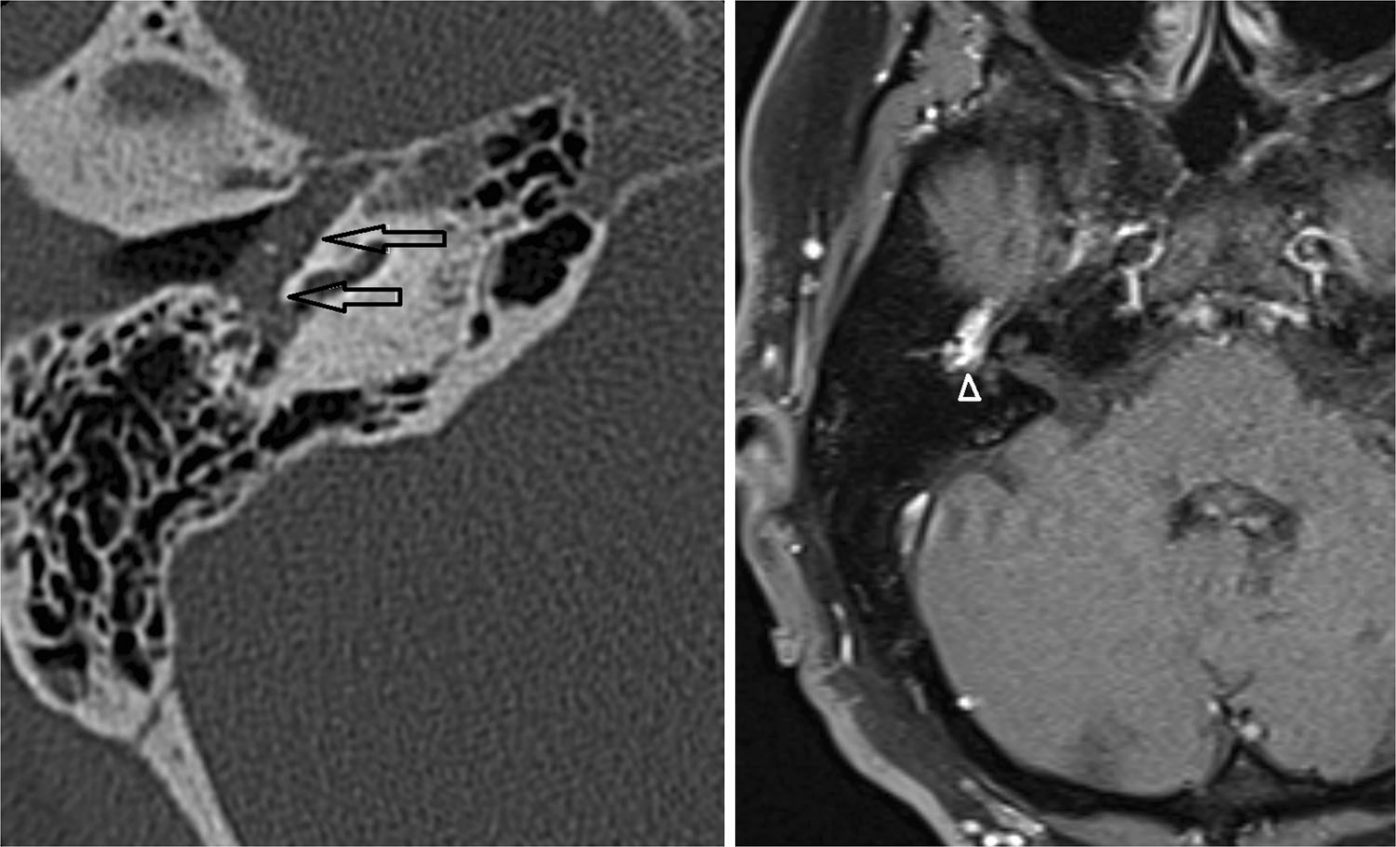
Glomus tympanicum. *Left* Axial CT shows a soft tissue mass in the middle ear (*arrows*). No visible bony erosion. *Right* Axial contrast-enhanced T1-W with fat suppression demonstrates strong enhancement of this lesion (*arrowhead*)
